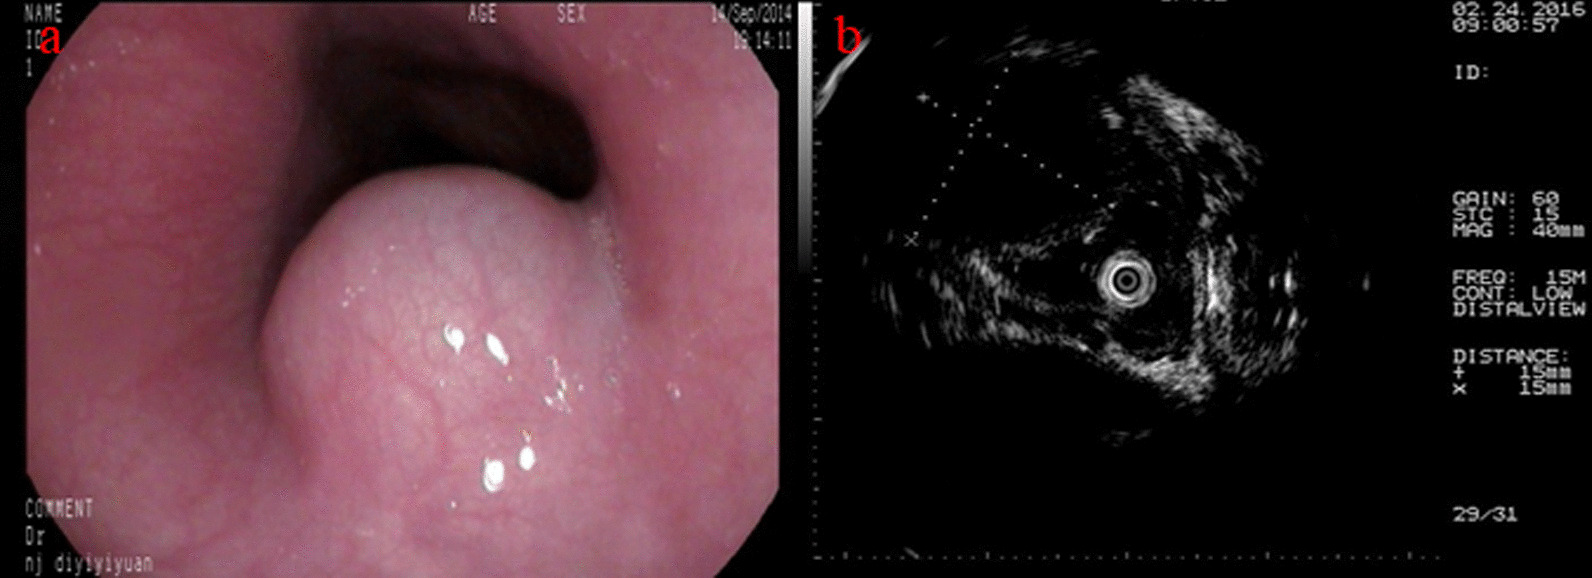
a Endoscopic image showing a 15 × 15 mm bulging lesion in the esophagus 30 cm from the incisors. b Endoscopic ultrasound revealed a hypoechoic cystic lesion arising from the muscularis propria
